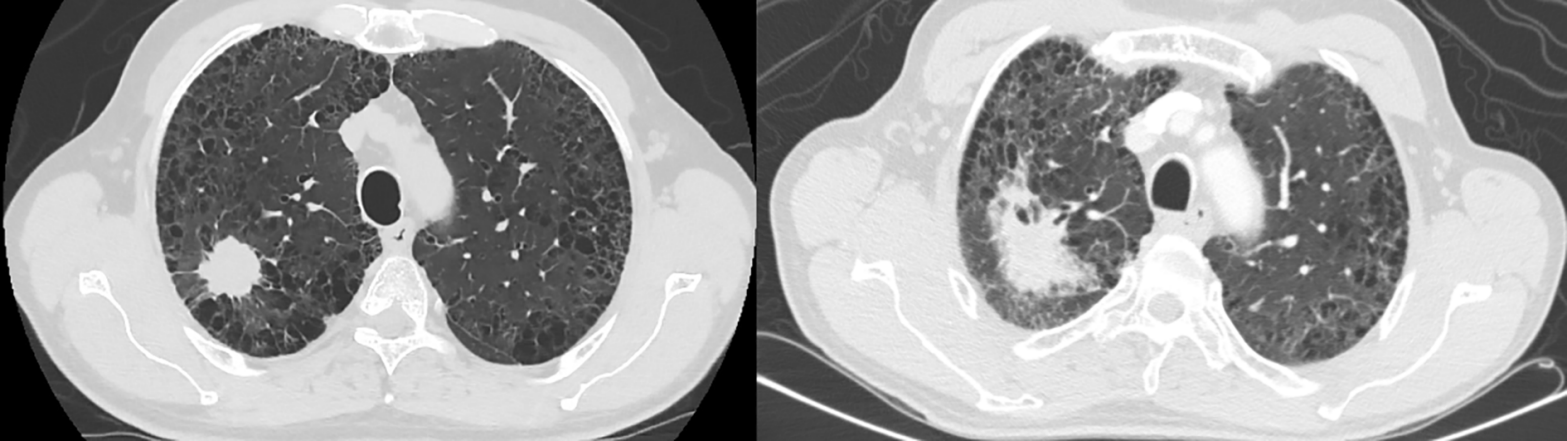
Pre‐treatment CT image from March 2021 (left) and the last CT image from November 2023 (right).
